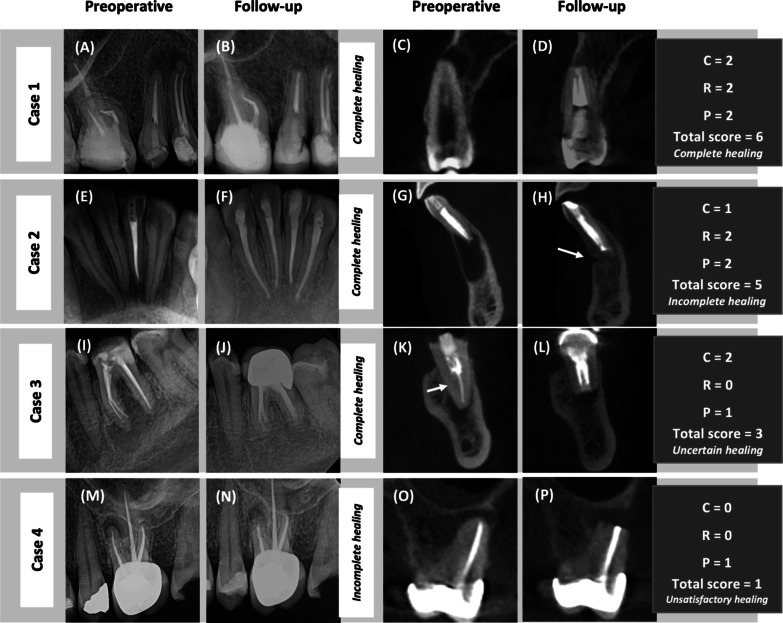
Preoperative and follow-up periapical radiographs (PAs) and cone-beam computed tomography (CBCT) images in corrected buccolingual views showing different patterns of radiographic healing. Case 1: (A–D): Maxillary second premolar presented at two-years after the surgery. (A and B) PAs showed complete healing. (C and D) Example of complete healing with respect to all three indices. Case 2: (E–H): Mandibular left central incisor that was followed up after one-year and eight-months. (E and F) Example of complete healing in PA. (G and H) Example of incomplete healing in CBCT. The arrow points to the cortical plate (C-index), which showed minor indentation and received a score of 1; meanwhile, the resection plane (R) and periradicular area (P) showed complete bone deposition, receiving a score of 2 each. Case 3: (I–L): Mandibular first molar at the one-year follow-up. (I) PA showing periapical radiolucency (PARL) associated with both mesial (M) and distal (D) roots. (J) Follow-up PA showed complete healing, but the density of the bone on the surgical side of the D root was lower than of the surrounding bone. (K) Preoperative CBCT showed PARL related to the D root of the first molar. The arrow points to the missed distolingual canal. (L) Follow-up CBCT showed complete re-establishment of the C-index (score 2) and partial improvement in the P-index (score 1), whereas no improvement was observed in the R-index (score 0). Case 4: (M–P) Maxillary first molar presented at one-year and six-months after the surgery with clinical signs and symptoms of failure. (M and N) PAs showing incomplete healing. (O) Preoperative CBCT of the mesiobuccal root with PARL. (P) Follow-up CBCT: the P-index reflects partial bone formation (a score of 1), but C- and R- indices reflect a lack of bone deposition (a score of 0 each)
